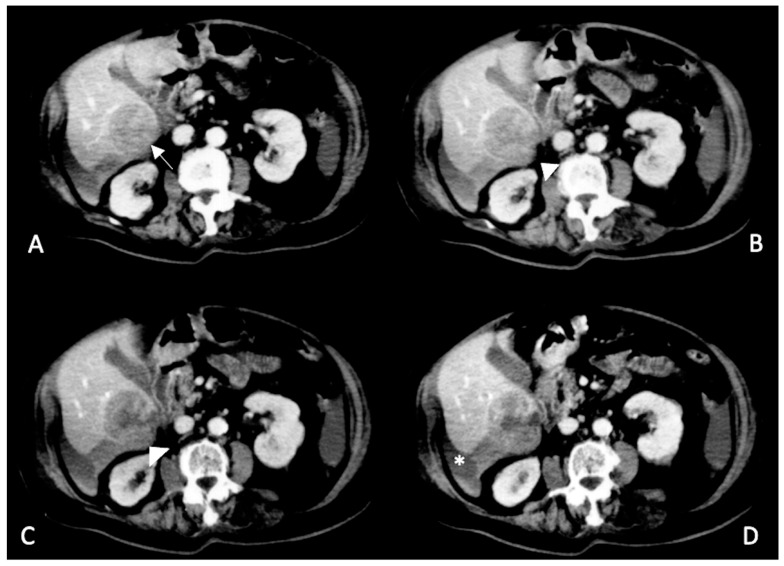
Arterial-phase enhanced CT scan. (A) Capsulated HCC of the VIII–V segment with inhomogeneous density (arrow). Note the disruption of the capsule with the consequent emptying of tumoral content (enucleation sign, arrowheads (B,C)). The hematocrit sign is also present ((D), asterisk).
